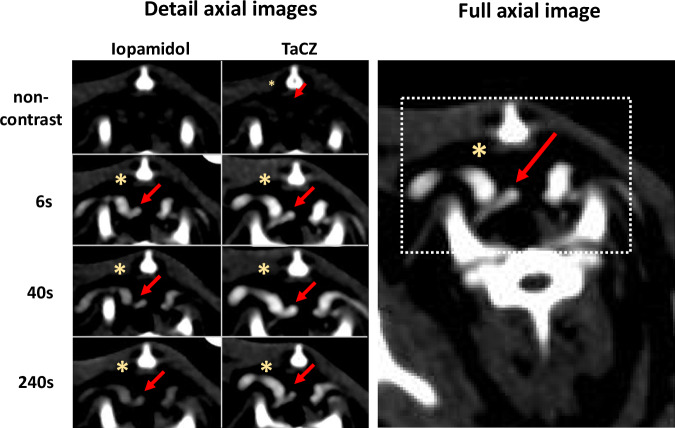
TaCZ improves the conspicuity of small arteries and veins at late contrast delays. Vascular contrast enhancement at the level of the thoracic aperture. The vascular ROI is enclosed by a white dotted rectangle (right image). Representative detail axial images (left) of 1.5 mm slice thickness display vascular contrast enhancement of the bilateral subclavian veins (yellow asterisk—right subclavian vein) at their confluence with the internal jugular veins. The brachiocephalic artery is highlighted by a red arrow, the left carotid artery is obscured by the vein. Images are displayed at CTA window levels (W/L = 300/170 HU) before (non-contrast), 6 s, 40 s, and 240 s after contrast administration (Iopamidol, left column; TaCZ, right column). TaCZ provides higher contrast enhancement of the shown vessels at all scan delays with sustained contrast at 240 s. CTA, Computed tomography angiography; L, Level; TaCZ, Carboxybetaine zwitterionic tantalum oxide; W, Width
